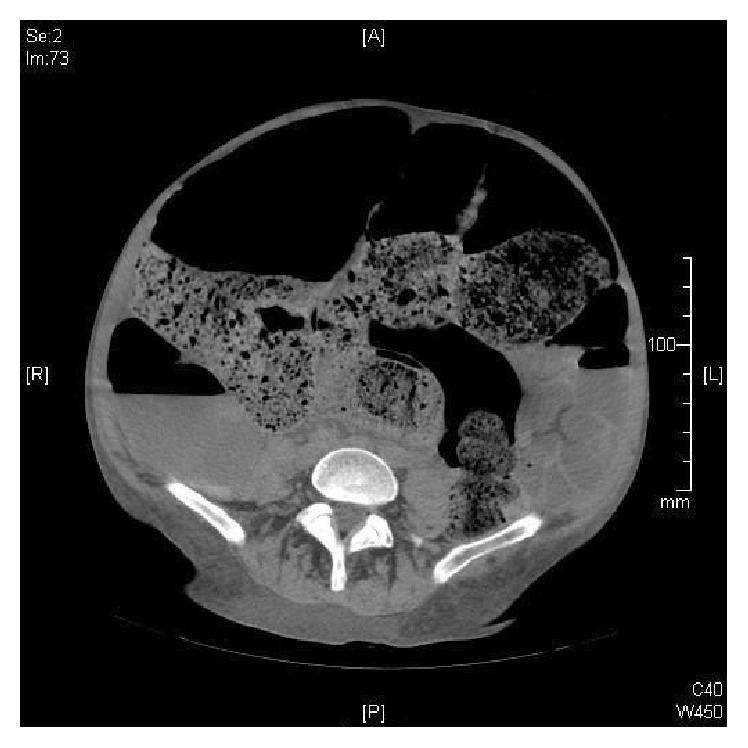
CT cut showing dilated colon and large stool burden.
